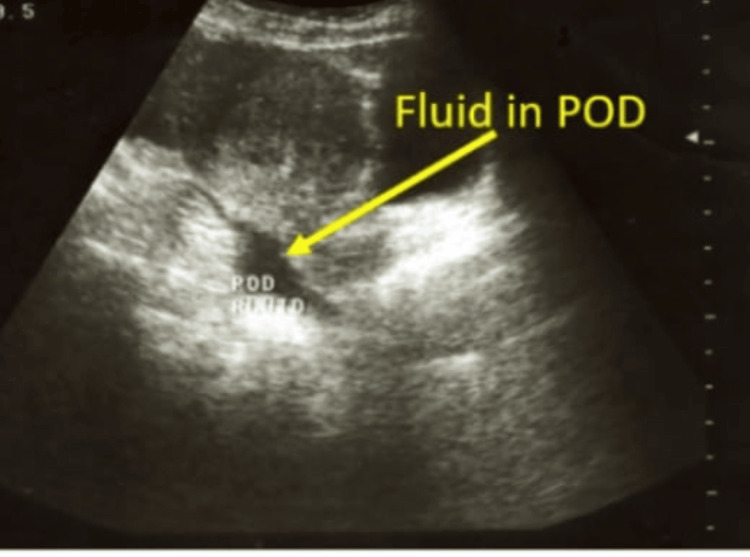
Tubal pregnancy seen on transvaginal sonography POD:  Pouch of Douglas
